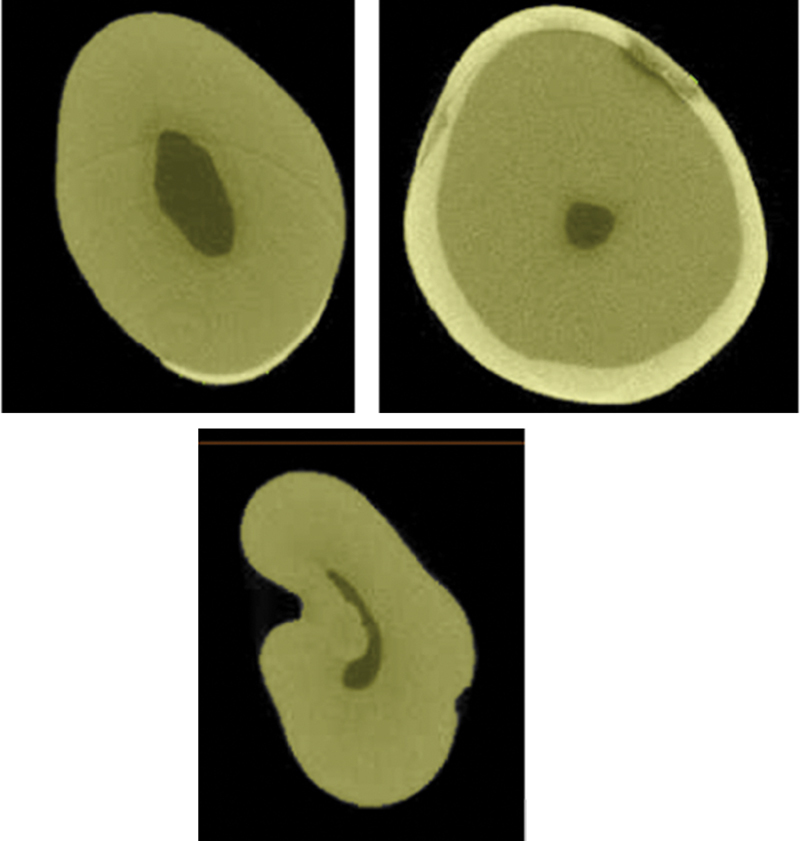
Microcomputed tomography images of the mandibular first premolar.
